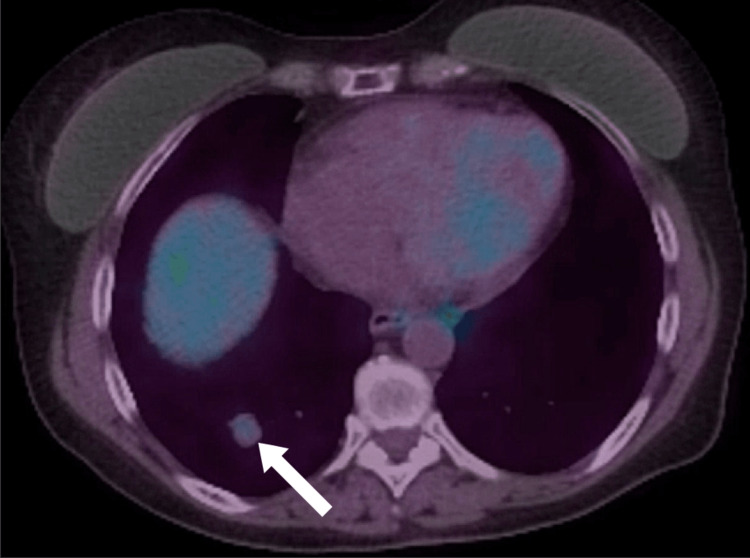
PET scan of right lower lobe lung nodule with trivial FDG-avidity (SUV 1.8)
